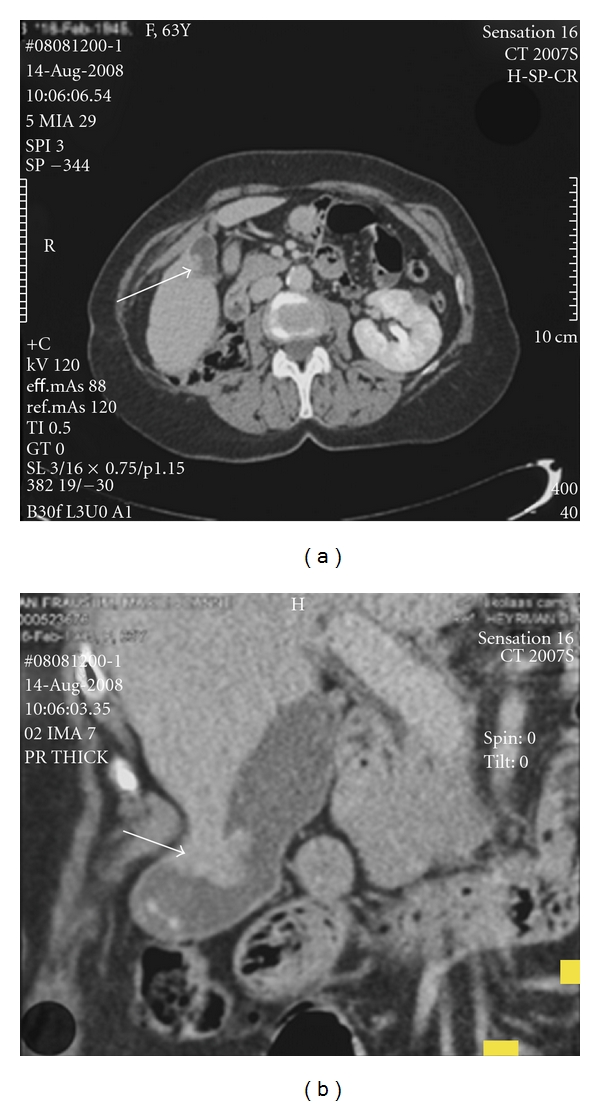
CT scan showing status after right nephrectomy and an evolutive papillary lesion in the gallbladder of approximately 1.9 cm ((a) and (b), arrows). (b) Contains the coronal reconstruction.
